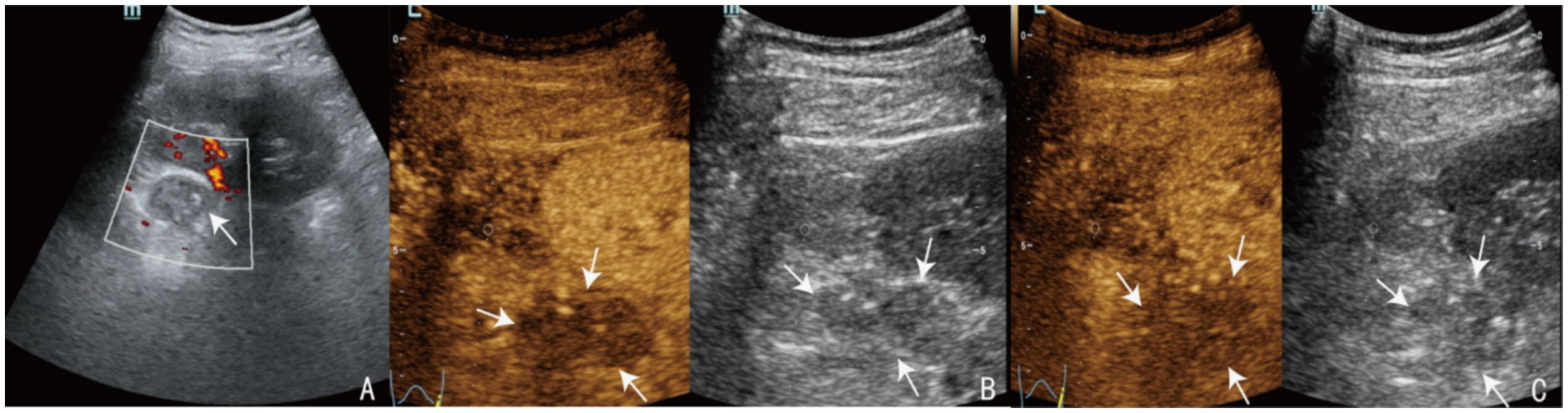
Two-dimensional ultrasound and contrast-enhanced ultrasound images of left adrenal tuberculosis. A hypoechoic nodule with calcification measuring approximately 2.7 × 2.2 cm was observed in the left adrenal region (A). Contrast-enhanced ultrasound demonstrates heterogeneous hypoenhancement of the nodule during both the corticomedullary and delayed phases (B,C) (the arrow indicates the lesion).
